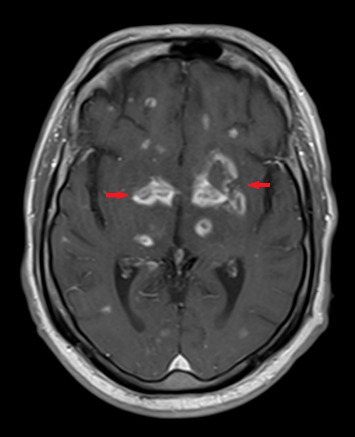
MRI of the brain with and without contrast reveals a worsening condition with an increase in both the number and size of lesions. There are numerous peripheral enhancing and nodular lesions throughout the cerebral and cerebellar hemispheres as well as the brainstem. The largest lesions (red arrows) are located in the deep gray nuclei, accompanied by increased edema.
